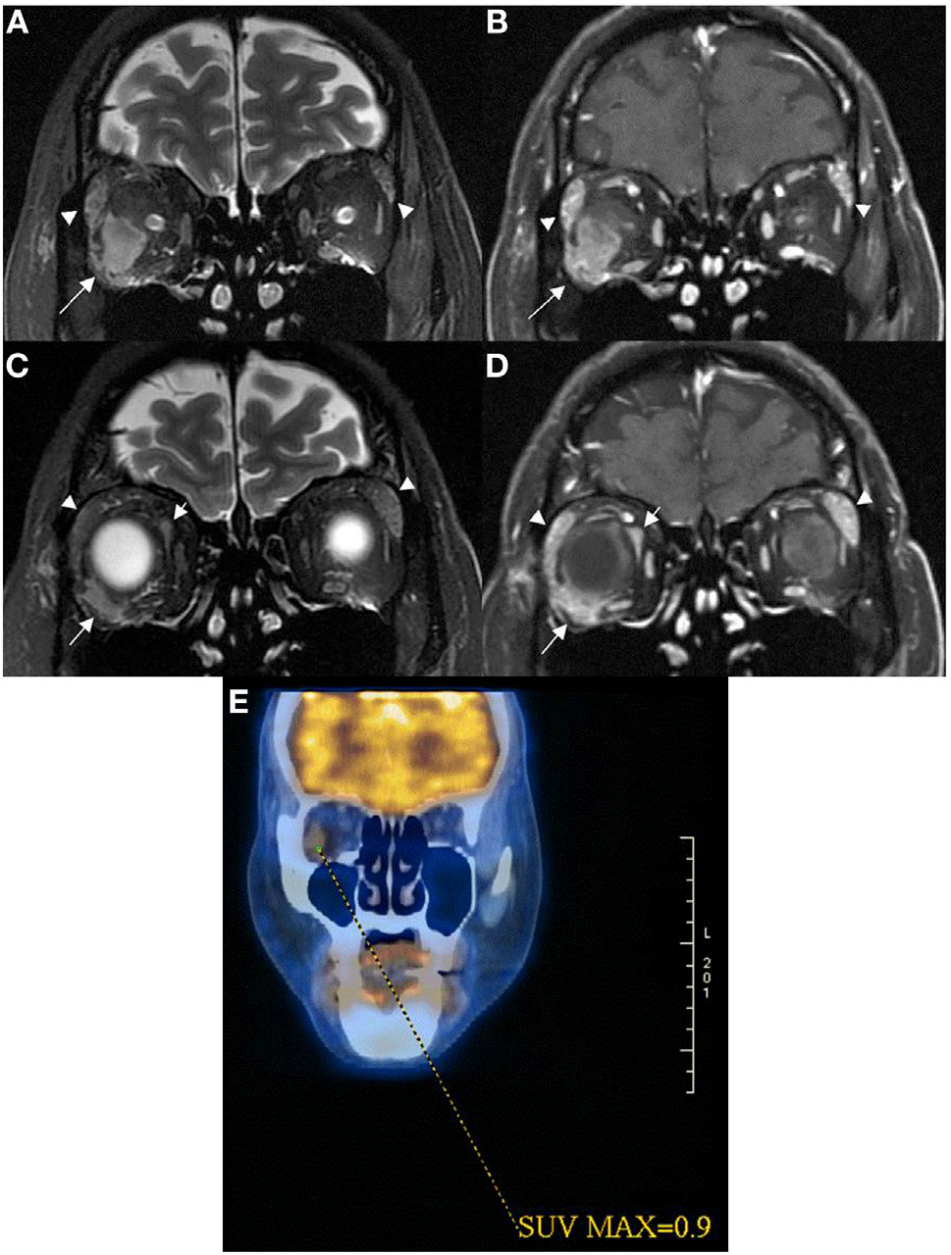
Coronal MRI of patient 2 showed intraconal orbital mass lesion (arrow) with low T2W signal (A) and modest contrast enhancement (B) having a 0.9 SUV on ^18^FDG PET image (E). Coronal MRI more anteriorly showed extraconal orbital lesions (short and long arrows) with low T2W signals (C) with modest contrast enhancement (D). One of the extraconal lesion infiltrated the outer lateral part of the right globe [long arrows in (C,D)]. Lacrimal glands were enlarged [arrowheads in (A–D)], and they demonstrated low T2W signals with modest contrast enhancement suggestive of lymphoid tissue infiltrate.
